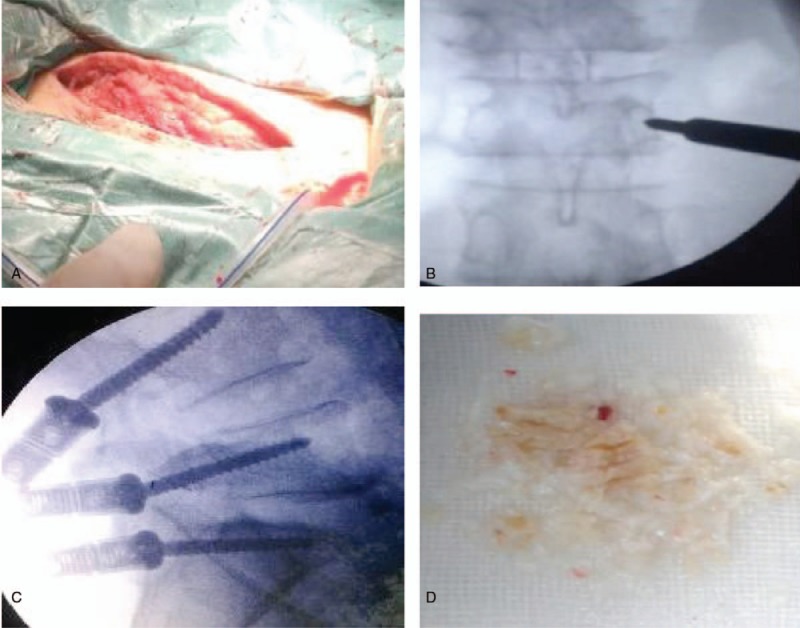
Sagittal T1-weighted MR images (left) and sagittal STIR MR images (right). (a and b) - Low signal intensity (SI) on T1-weighted images and high SI on STIR images of the endplates at L5-S1 related to a type I endplate change, (c and d) – High signal intensity on T1-weighted images and low signal intensity on STIR images of the endplates at L4-L5 related to type II endplate change.
